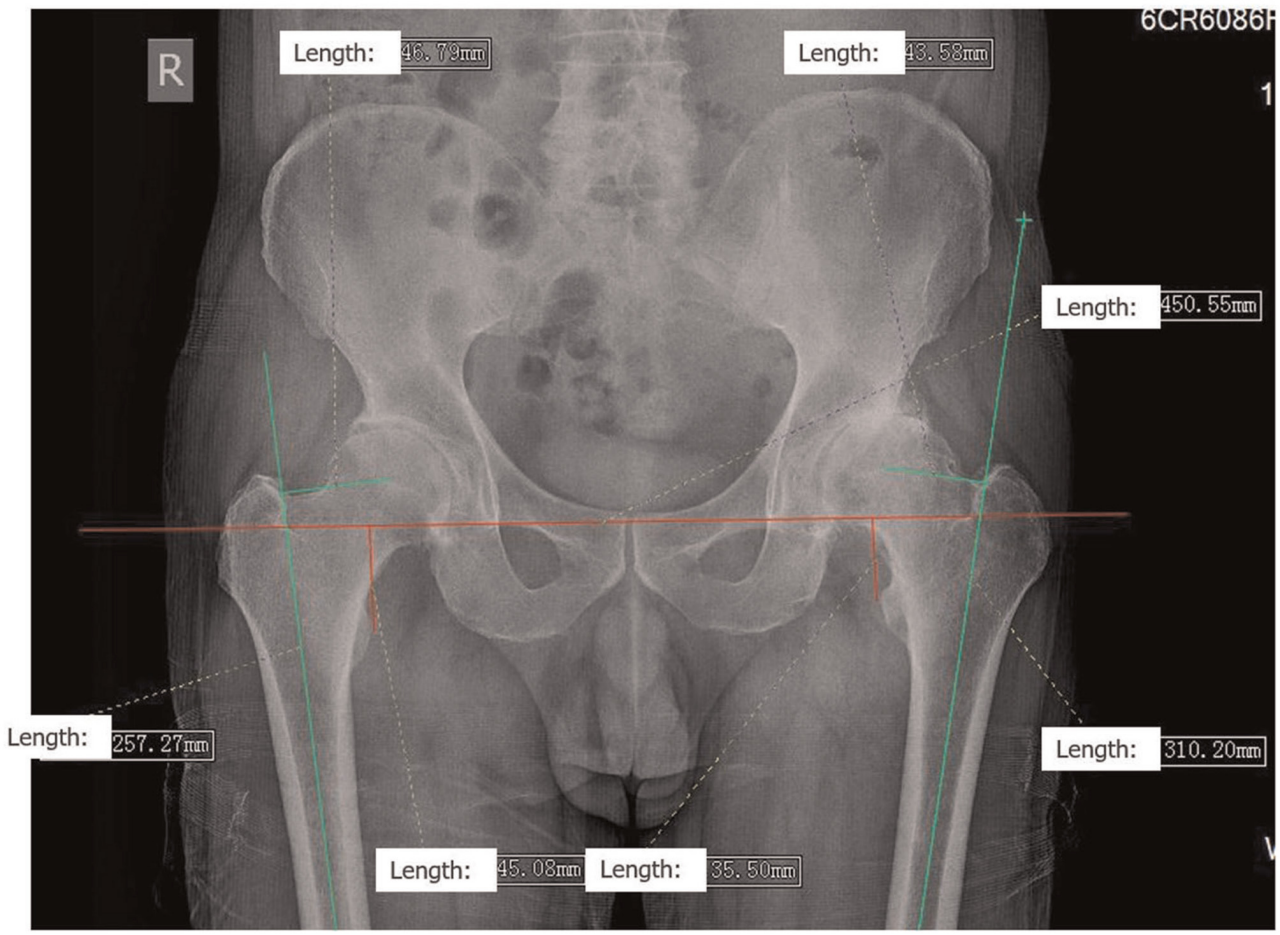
Preoperative X-ray templating All data measurements were made on Neusoft PACS. An anteroposterior (AP) positive view of the pelvis was obtained with both lower extremities internally rotated at 15°. For LLD measurement, a reliable method is to measure the vertical distance from the line connecting the lower edge of the two teardrops to the innermost edge of the small rotor. The difference between the measured values of the two sides is the LLD. OD is measured by the distance between the axis of the femur and the center of the femoral head. In the figure, the LLD was 9.58 mm, the OD on the right was 46.79 mm, and the OD on the left was 43.58 mm.
